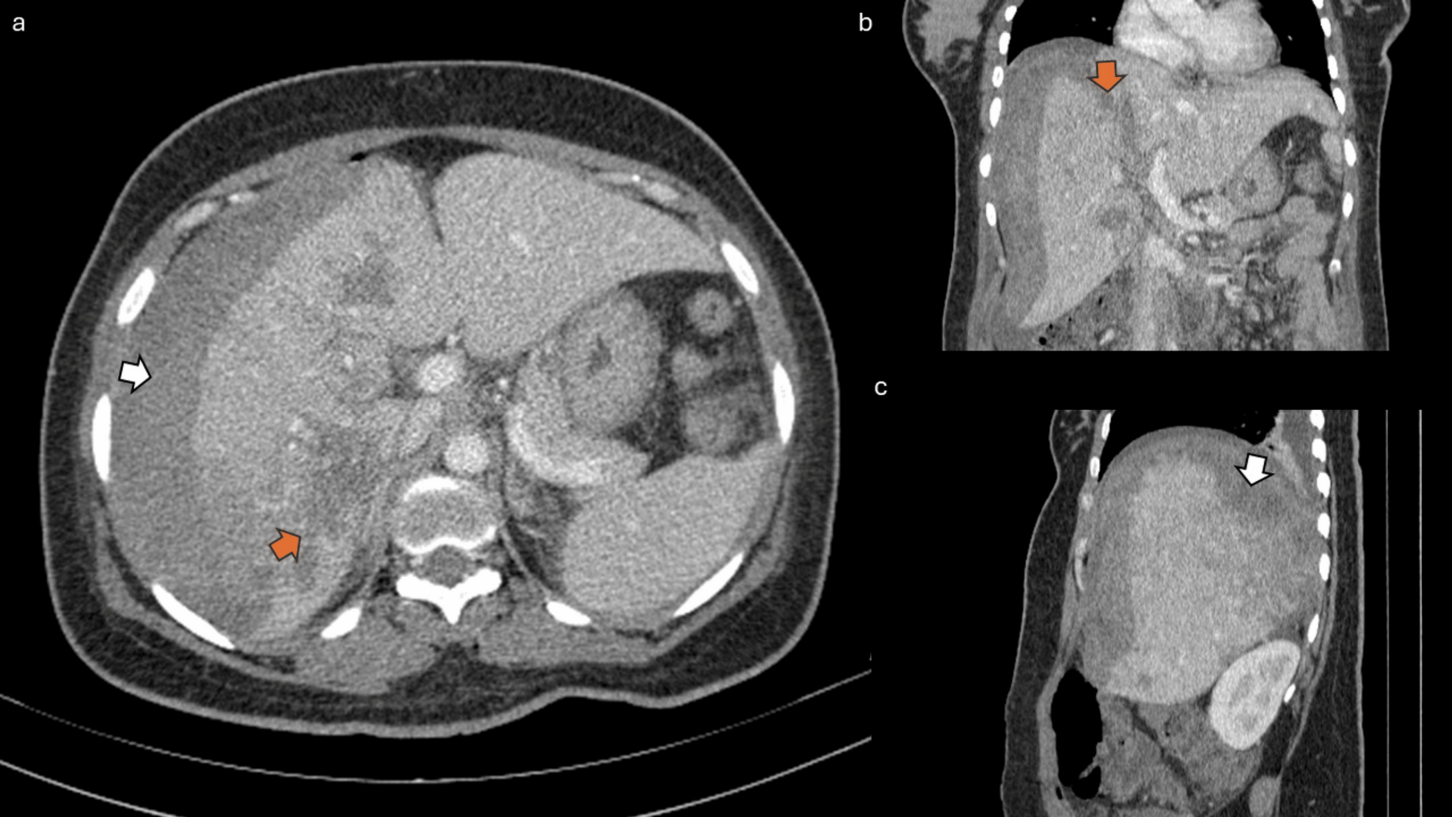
Contrast-enhanced CT scan of the abdomen in axial (a), coronal (b), and sagittal (c) views revealing a heterogeneous subcapsular hepatic hematoma (white arrows) over the right hepatic lobe and multiple poorly defined hypodense areas of hepatic infarction (orange arrows). CT: computed tomography
